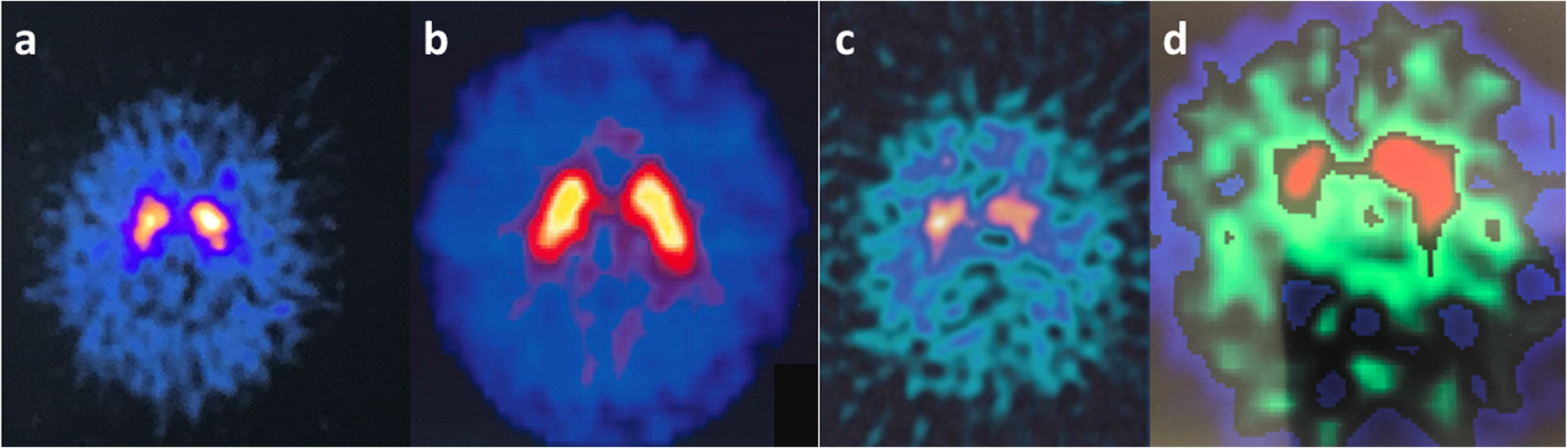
^123^I-Ioflupane SPECT imaging, a measure of dopamine transporter density, demonstrates in case 2 normal radiotracer uptake or very mild hypoactivity in right caudate (A); in case 3 normal radiotracer uptake bilaterally (B); in case 6 bilateral striatal hypoactivity (C) and in case 8 bilateral hypoactivity with a more marked reduction in right striatum(D)
